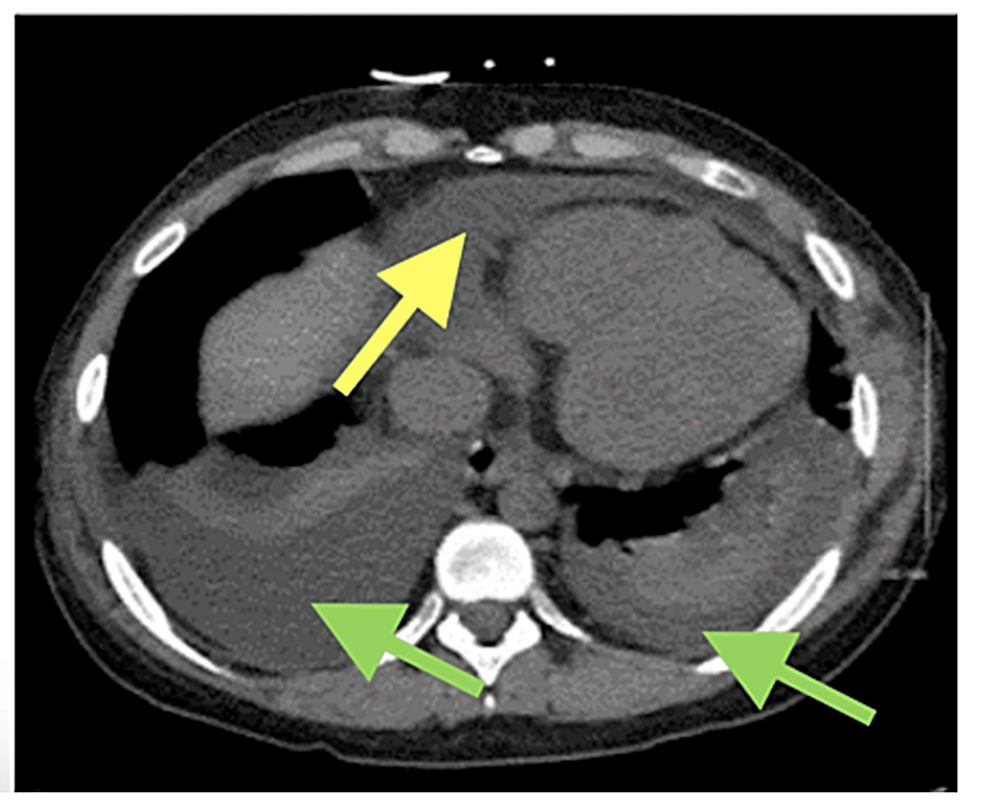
CT scan without contrast shows the recurrence of cardiac tamponade three weeks after Yellow arrows indicate pericardial effusion; Green arrows indicate pleural effusions
